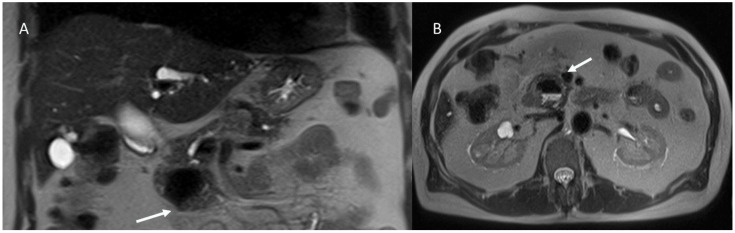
MRI of a 68-year-old male patient with Todani type II lesion. White arrows in coronal (A) and axial (B) T2-weigthed sequences show an extrahepatic supraduodenal diverticulum.
